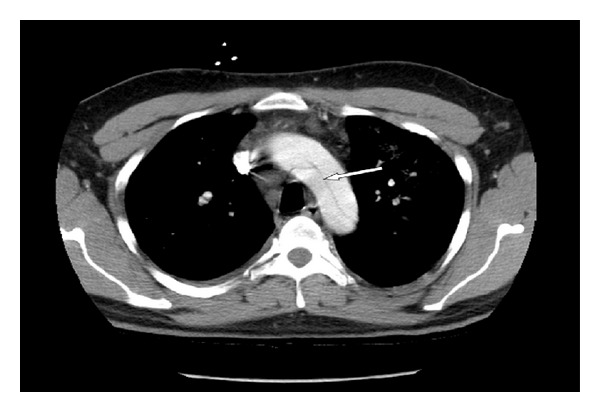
CT scan of chest prior to admission shows aortic dissection.
